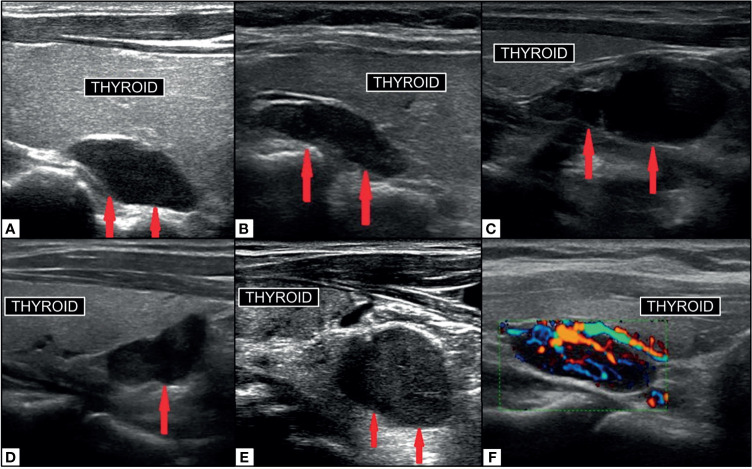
Ultrasound imaging of parathyroid adenoma (red arrows). Right superior PA (A) in median retrothyroidal location, hypoechoic, measuring 2.2cm. Left superior PA (B) in the upper retrothyroidal location, hypoechoic, measuring 2.3 cm. Predominantly cystic right inferior parathyroid adenoma (C) adjacent to lower pole of thyroid, anechoic, measuring 3.5 cm. Right inferior PA (D) in the lower retrothyroidal location, hypoechoic, measuring 2 cm. Left inferior PA (E) near the lower pole of thyroid extending to the retrosternal region, hypoechoic, measuring 2.3 cm. PA on color-doppler ultrasonography (F) with central hyperemia and afferent ‘polar’ vessel. Reproduced with permission from: *Vitetta GM, Ravera A, Mensa G, Fuso L, Neri P, Carriero A, Cirillo S. Actual role of color-doppler high-resolution neck ultrasonography in primary hyperparathyroidism: a clinical review and an observational study with a comparison of 99mTc-sestamibi parathyroid scintigraphy. J Ultrasound. 2019 Sep;22(3):291-308. doi: 10.1007/s40477-018-0332-3. Epub 2018 Oct 24. PMID: 30357759; PMCID: PMC6704209*.
